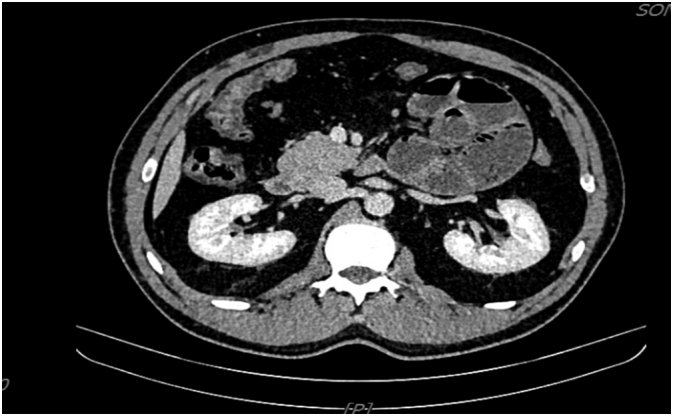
Axial contrast-enhanced abdominal computed tomography (CT) images of the left paraduodenal hernia in a 39-year-old man.
